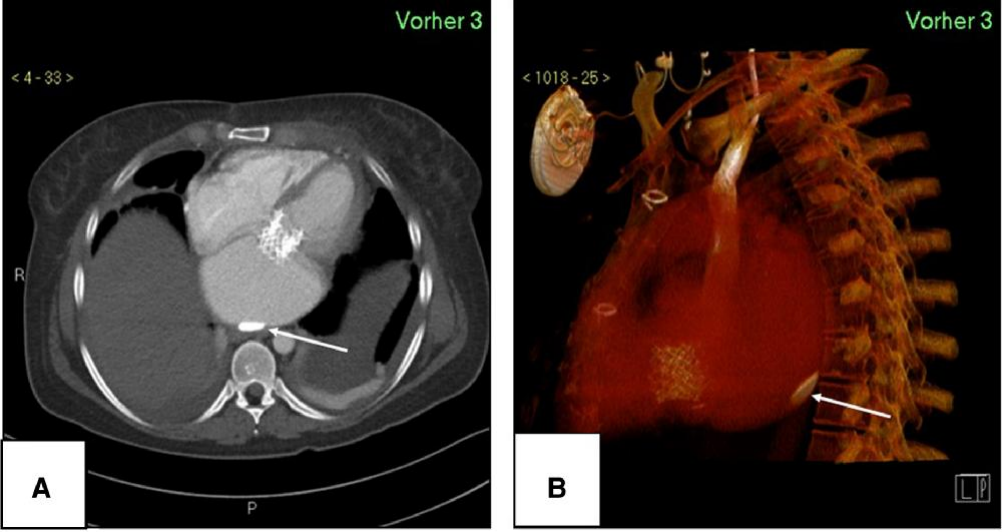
Chest CT taken in supine position in transverse plane (*A*) and sagittal plane (*B*) showing the tilt (white arrow) in the inferior-posterior left atrium.
